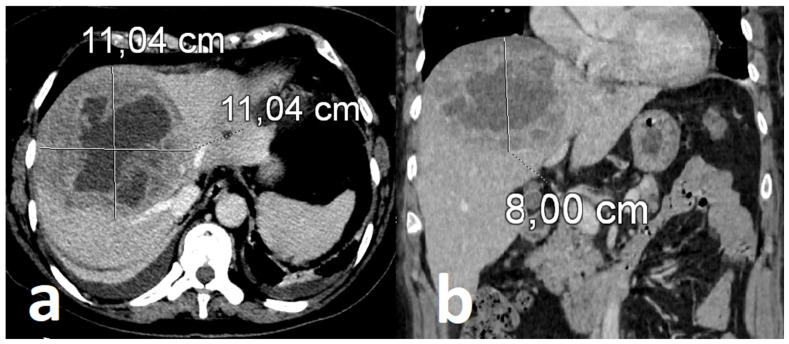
Radiological progression of hepatic abscess measuring approx. 11 × 11 × 8 cm in follow-up CT scan: axial (a) and coronal (b) plane views.
